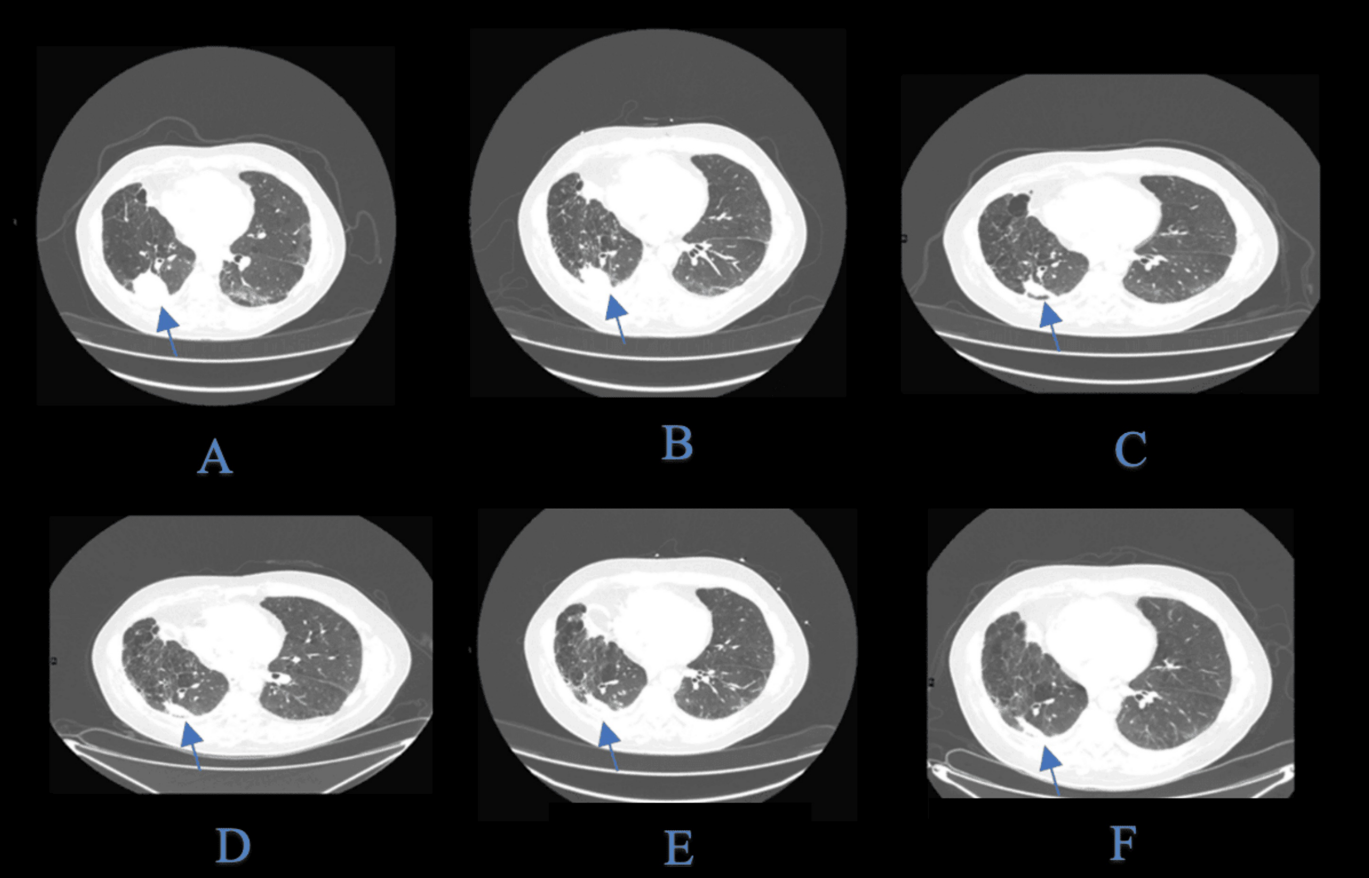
Sequential CT images of the lung index lesion. Similar pattern of regression was noted in the liver metastasis without the development of new lesions (arrows). An initial CT scan in April 2022 revealed marked metastatic disease in the right lung and liver prior to treatment. After chemotherapy and a single dose of pembrolizumab in August 2022, early tumor regression was observed. By November 2022, further shrinkage of the metastatic lesions was noted, with no new sites identified. In March 2023, there was continued regression of pulmonary and hepatic disease, reflecting a deepening response. By January 2024, radiographic imaging showed minimal disease. As of November 2024, over 20 months post-ICI, the disease remained stable, with no progression or new metastases, and no additional therapy required. ICI: immune checkpoint inhibitor
